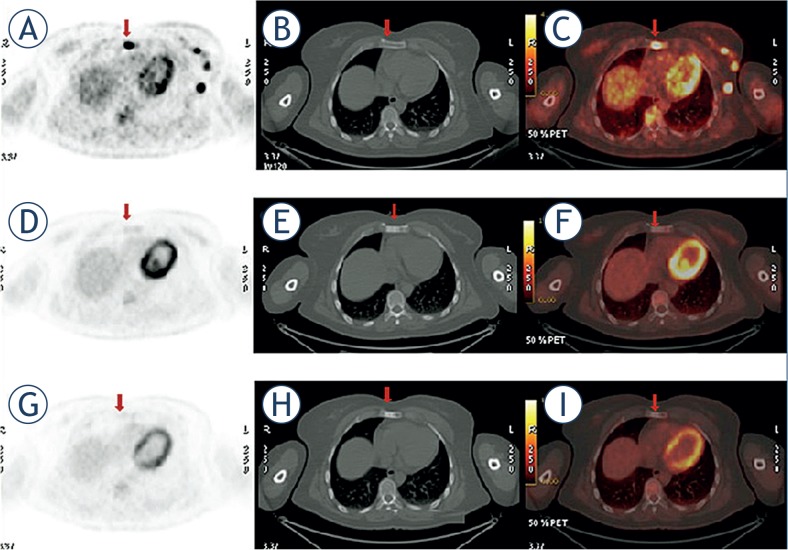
Baseline CT-negative ^18^FDG-positive bone marrow metastasis is becoming sclerotic (CT-positive) while decreasing metabolic activity (^18^FDG-negative) as a response to therapy. Baseline transaxial (A)
